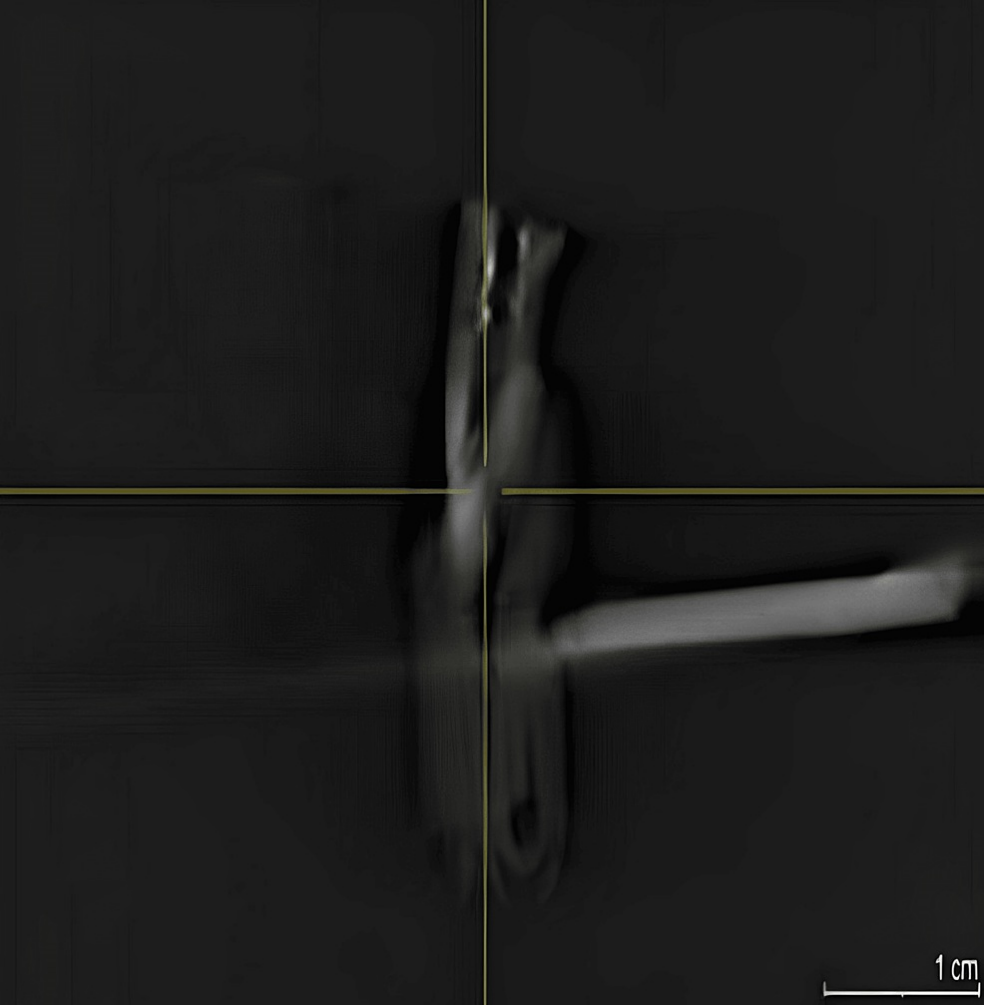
CBCT image after retreatment CBCT, cone beam computed tomography
